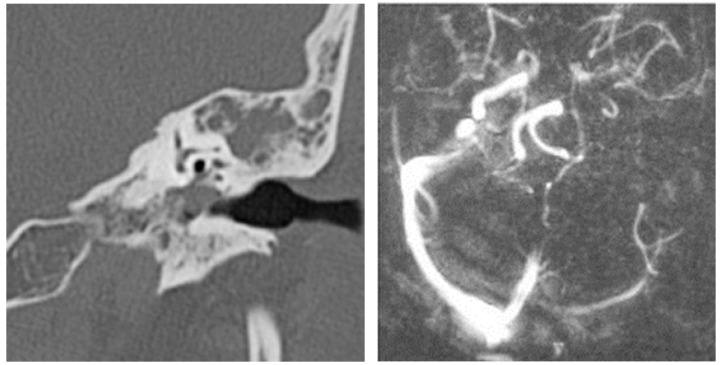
Patient with diagnosis of chronic suppurative otomastoiditis, lateral venous sinus thrombosis. CT scan, axial section, bony window showing absence of pneumatization of left-sided mastoid cells, fluid retention in the area, and MR venous 2DTOF MRI venous 2DTOF sequence with absence of signal at the left-sided lateral venous sinus.
